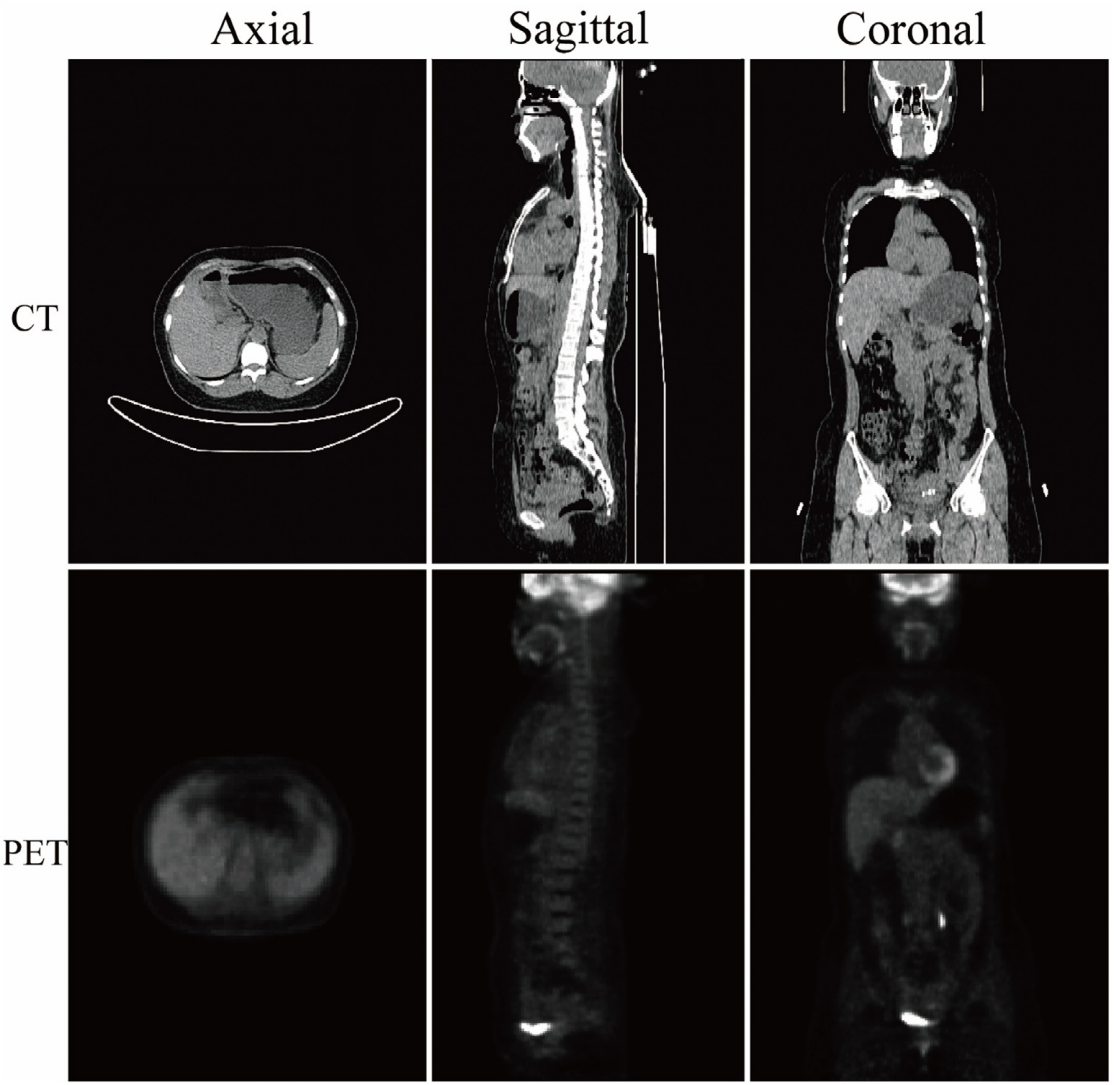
Example of the whole-body PET/CT images. The first and second rows correspond to the CT and PET images, respectively. The first to the third columns correspond to the axial, sagittal and coronal views of the 3D volume data, respectively.
